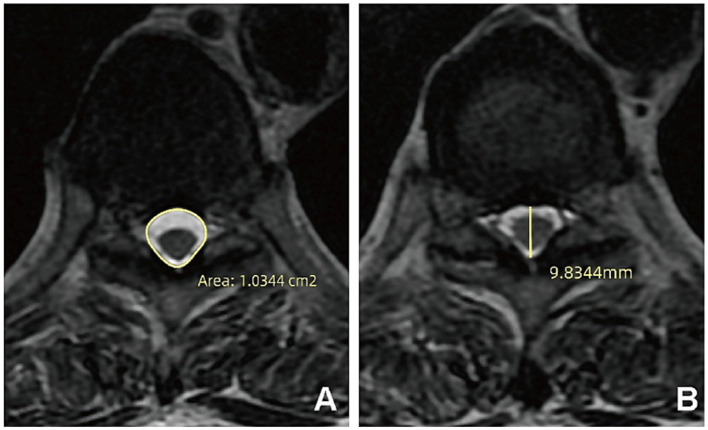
Measurement of cross-sectional area (A) and anteroposterior diameter (B) on preoperative and postoperative MRI.
